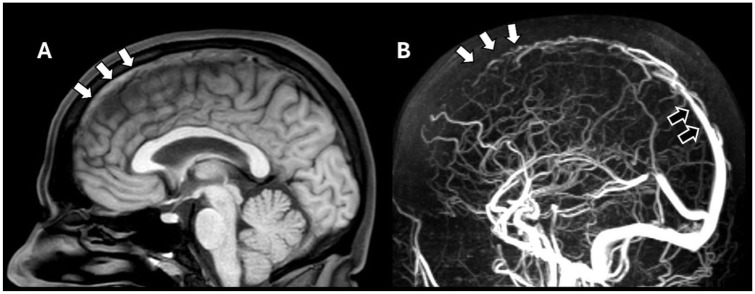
(a) Mid-sagittal T1 weighted MRI showing hyperintensity in the anterior superior sagittal sinus, indicating fresh thrombus within the venous sinus (white arrows). (b) Sagittal MR-venogram of the same patient. There is absence of flow within the anterior superior sagittal sinus, confirming there is a thrombus in this vein (white arrows). For reference, normal opacification is seen in the posterior segment of the superior sagittal sinus (black arrow).
